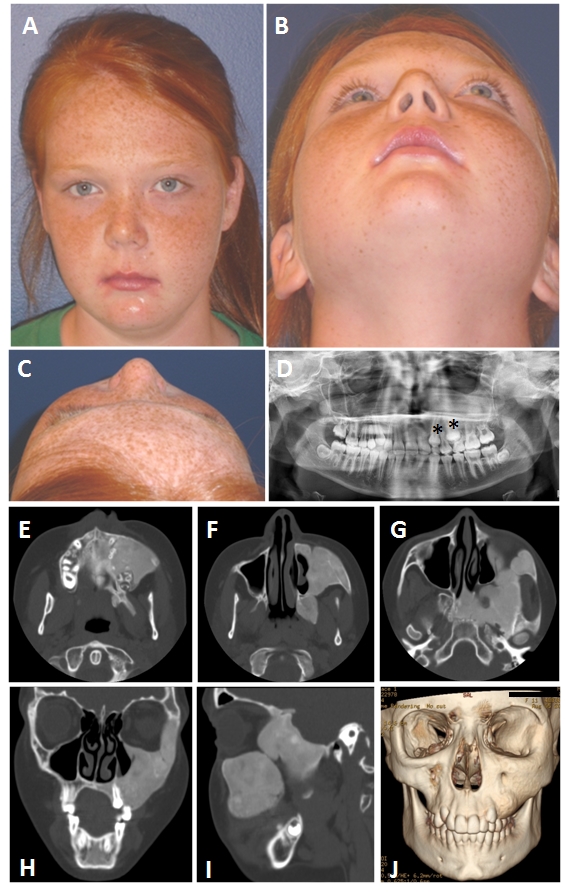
An 11-year old female with monostotic fibrous dysplasia of the left zygomatic-maxillary region. A-C) Clinical photographs demonstrating the appearance. The lesion was quiescent and asymptomatic. It had grown slowly over a period of years. D) Her dentist noted delayed eruption of her teeth (*) on that side as well as mild facial asymmetry and obtained the panorex that identified the lesion. E-I) CT images demonstrate the pathognomonic appearance of FD for her age, a homogenous, “ground-glass” lesion. J) The reconstructed CT image gives a sense of the three dimensional shape of the lesion that accounts for the clinical appearance.
